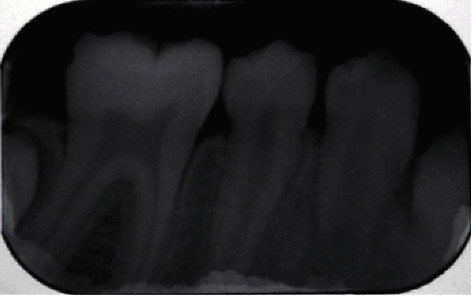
Twelve-month postoperative radiograph showing spontaneous eruption of the tooth 44.
